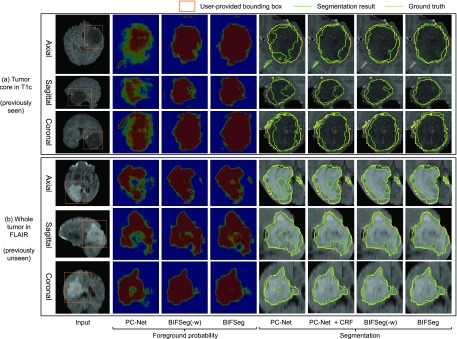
Visual comparison of PC-Net and unsupervised refinement methods without additional scribbles for 3D brain tumor segmentation. The same initial segmentation obtained by PC-Net is used by different refinement methods. (a) Tumor core in T1c (previously seen). (b) Whole tumor in FLAIR (previously unseen).
